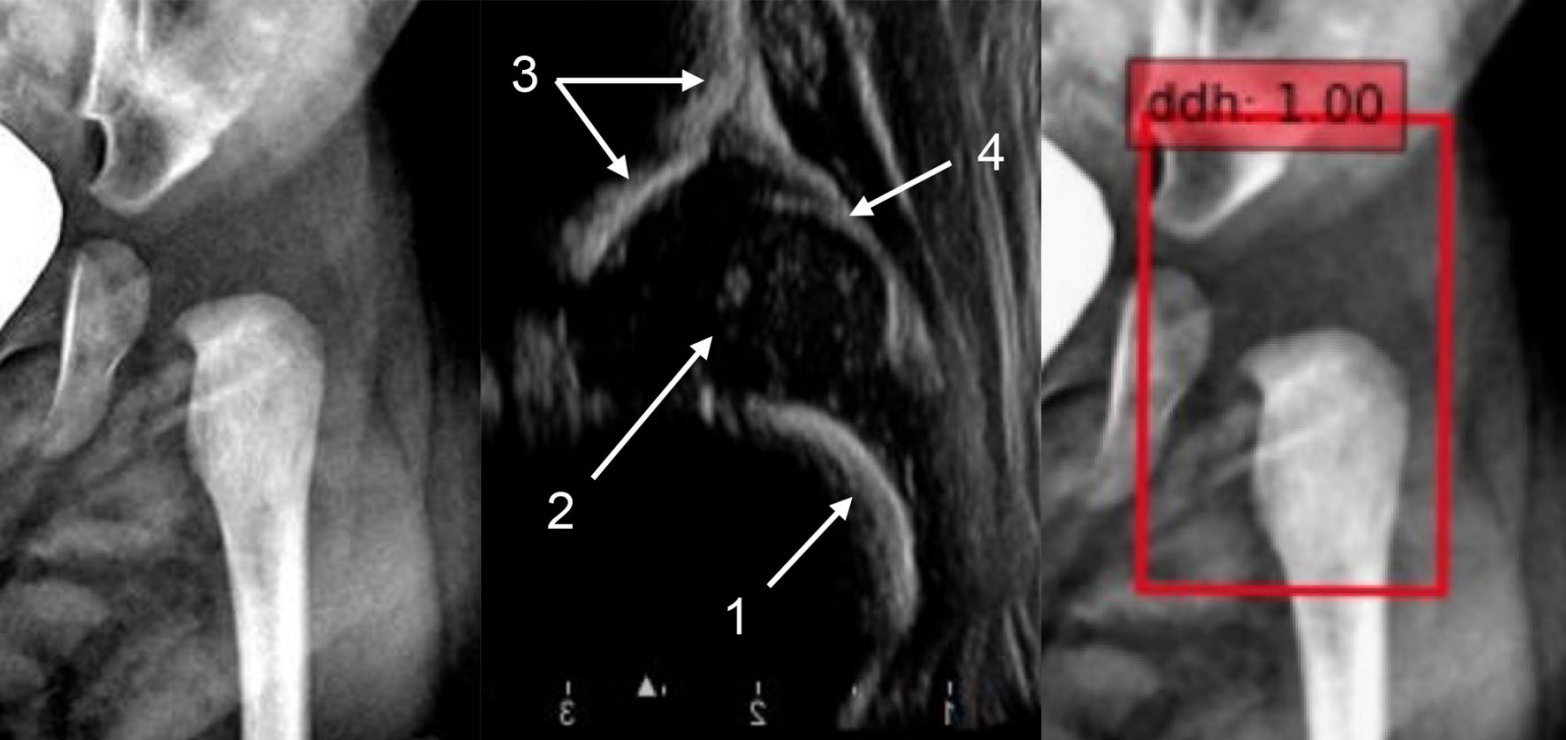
Hip anteroposterior radiography image (left), hip ultrasonography image of the same hip (middle), and the SSD model image output (right). The patient was a 1-month-old boy. IHDI grade 2, Graf type D. Anatomical interpretation of the ultrasonography image: 1, bony part of the femoral neck. 2, cartilaginous femoral head. 3, bony part of acetabular roof. 4, acetabular labrum. *IDHI* International Hip Dysplasia Institute, *SSD* Single shot multi-box detector.
